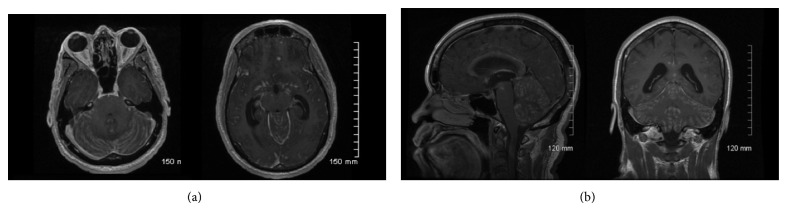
Initial MRI: T1 postcontrast axial images on the top row, sagittal and coronal on bottom row.
